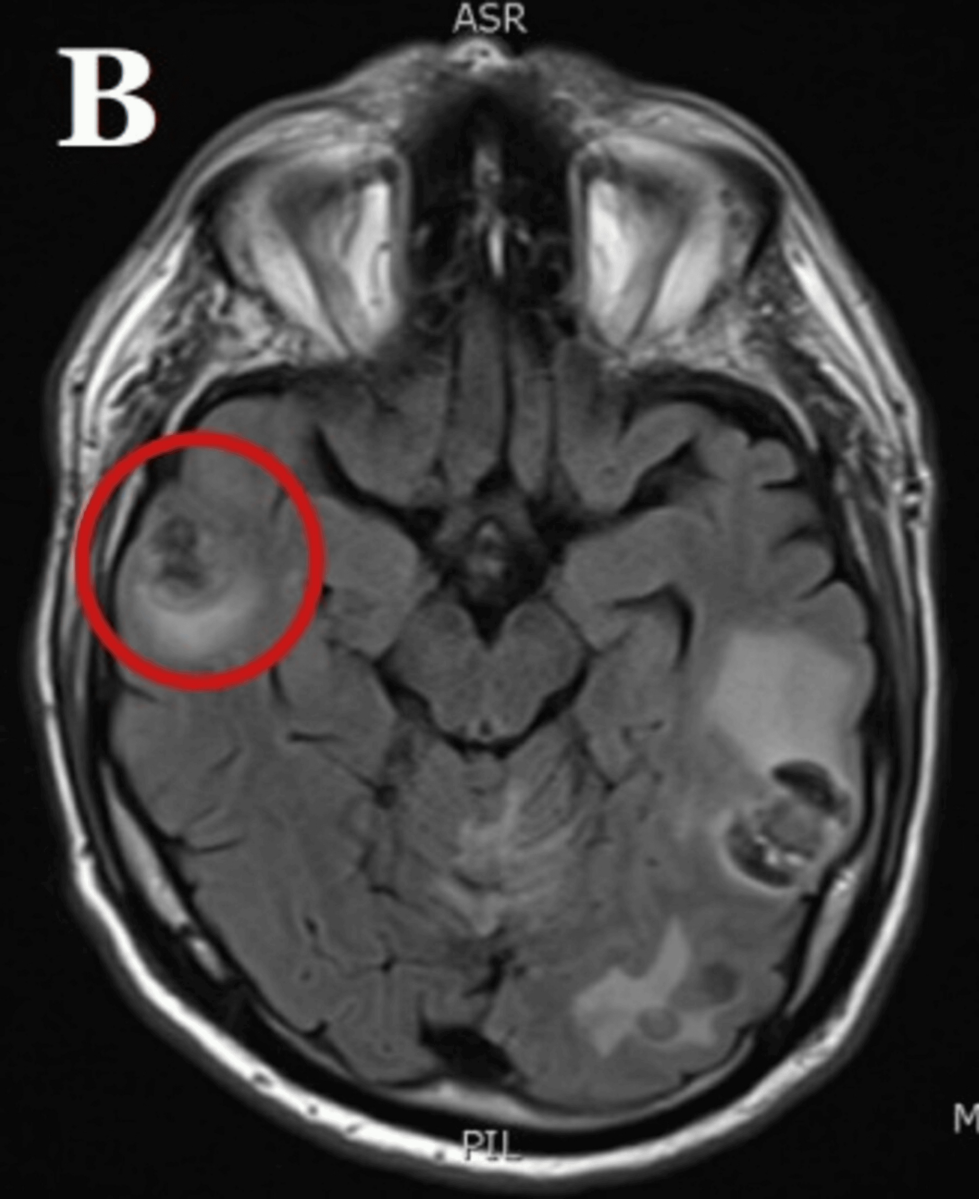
MRI brain showing a 1.4 x 1.0 cm lesion in the right lateral ventricle frontal horn and associated vasogenic edema surrounding the lesions
